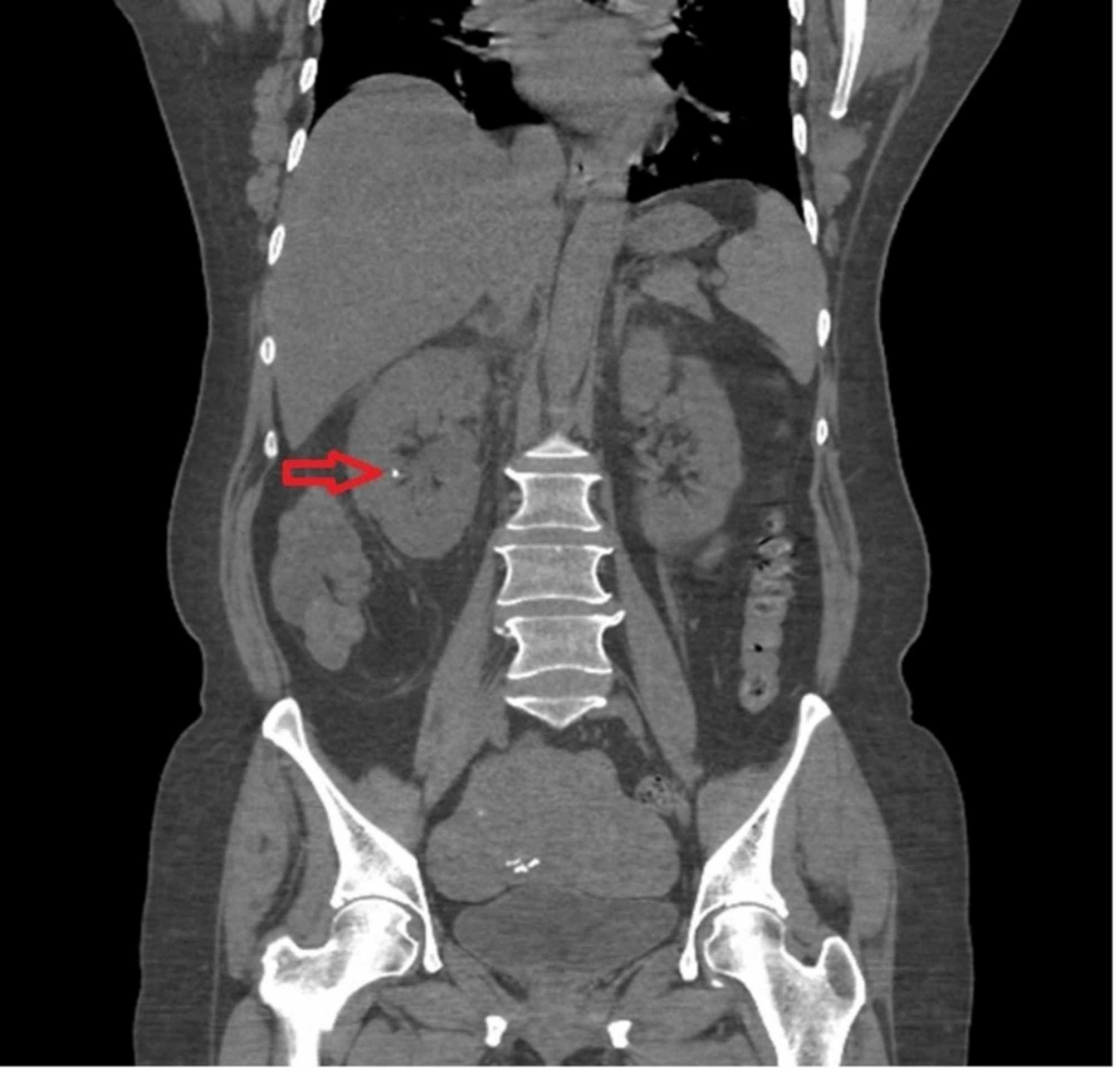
Computed tomography of the abdomen with contrast showing non-obstructive right renal calculi (red arrow)
